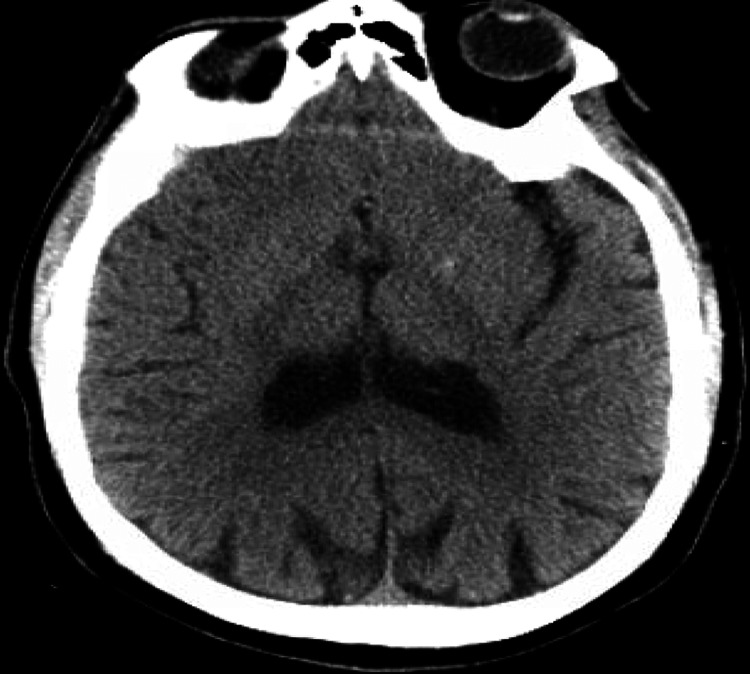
Initial CT head without contrast: no cerebral edema.
